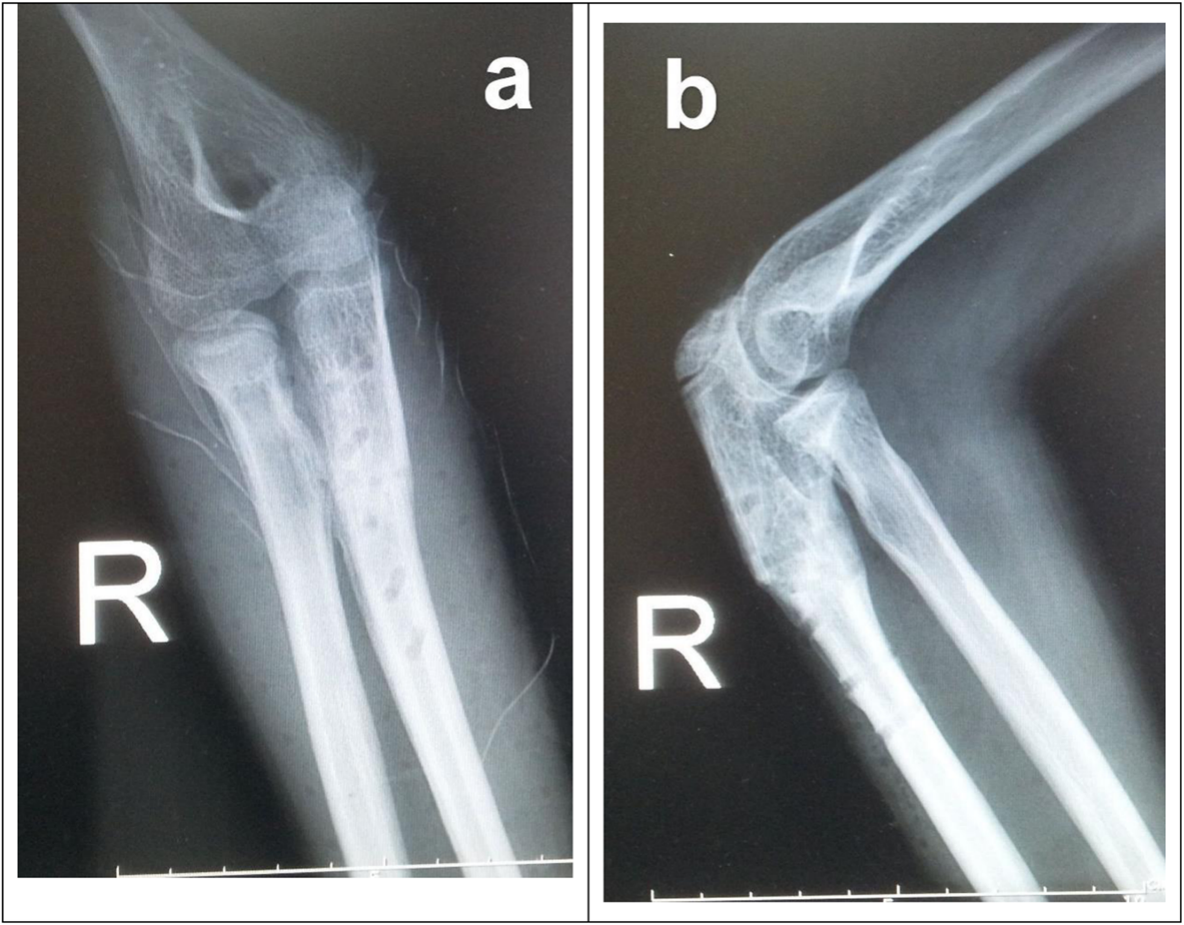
a Final AP X-rays at one-year follow-up showing bony union in the radial and ulnar osteotomy sites with excellent radiocapitellar congruity and proximal ulnar shaping. b Final lateral X-rays at one-year follow-up showing bony union in the radial and ulnar osteotomy sites with excellent radiocapitellar congruity and proximal ulnar shaping
